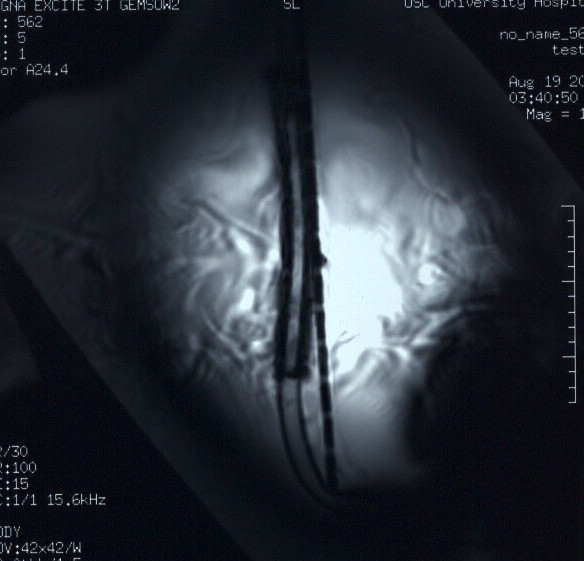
MRI artifact associated with the prototype Nitinol wire-reinforced atrial cannula used with the AB5000 Ventricle (gradient echo pulse sequence; TR/TE, 100-msec/15-msec; flip angle, 30 degrees; matrix size, 256 × 256; section thickness, 10-mm; field of view, 42-cm; long axis imaging plane).
